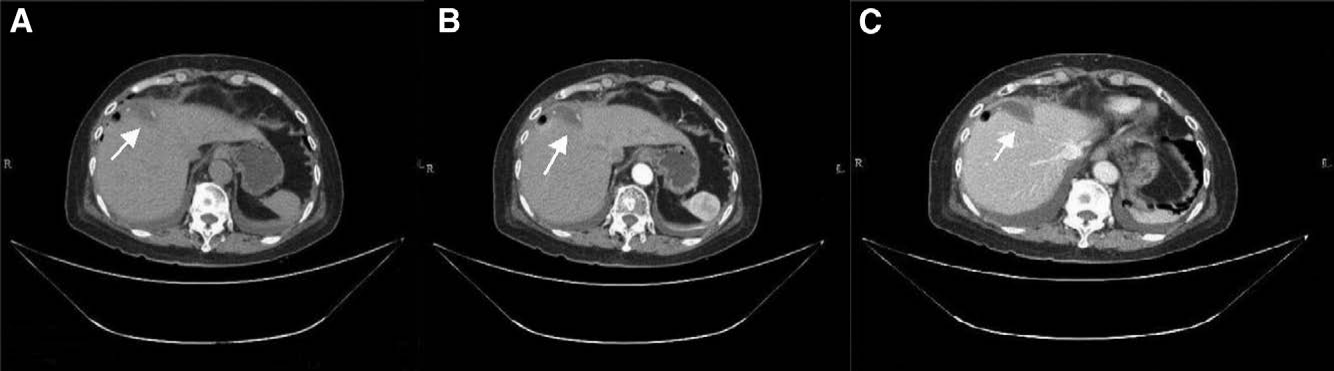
Preoperative CT images. (A) Plain CT scan showing low-density circular nodules in the left anterior lobe of the liver. (B) CT arterial phase enhancement showed mild enhancement. (C) CT portal phase enhancement showed a mild degree of enhancement, which was low density relative to normal liver tissue. CT = computed tomography.
